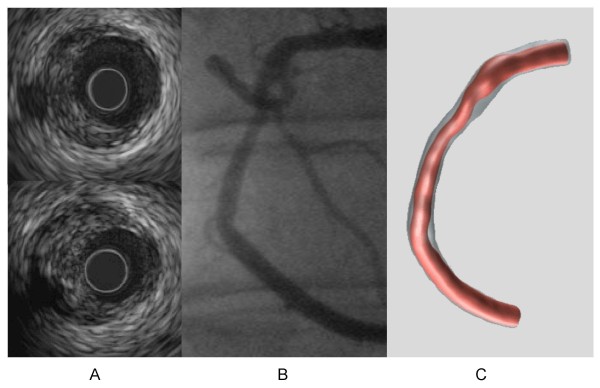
The intravascular ultrasound images of a mildly stenosed right coronary artery (panel A), the corresponding contrast angiogram (panel B) and the 3D reconstruction (panel C).
